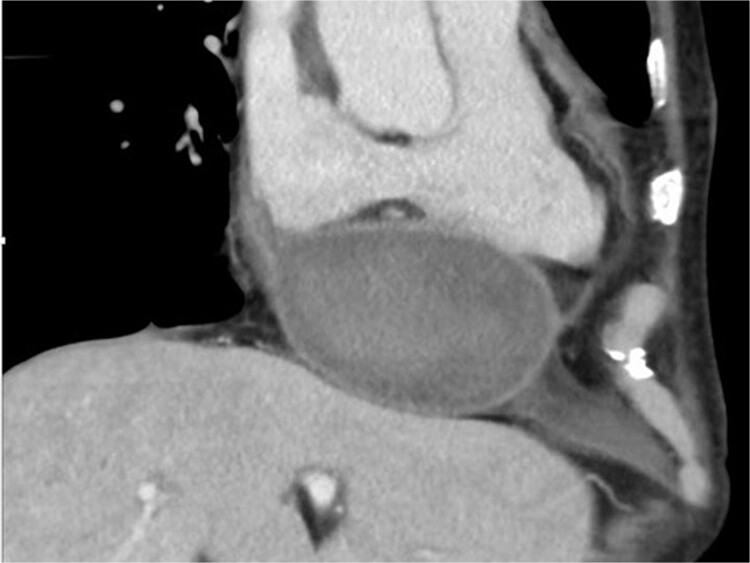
Computed tomography scan at 2 months after left ventricular rupture repair. There is a huge pseudoaneurysm (110 × 57 mm) on the posterior side of the left ventricle.
